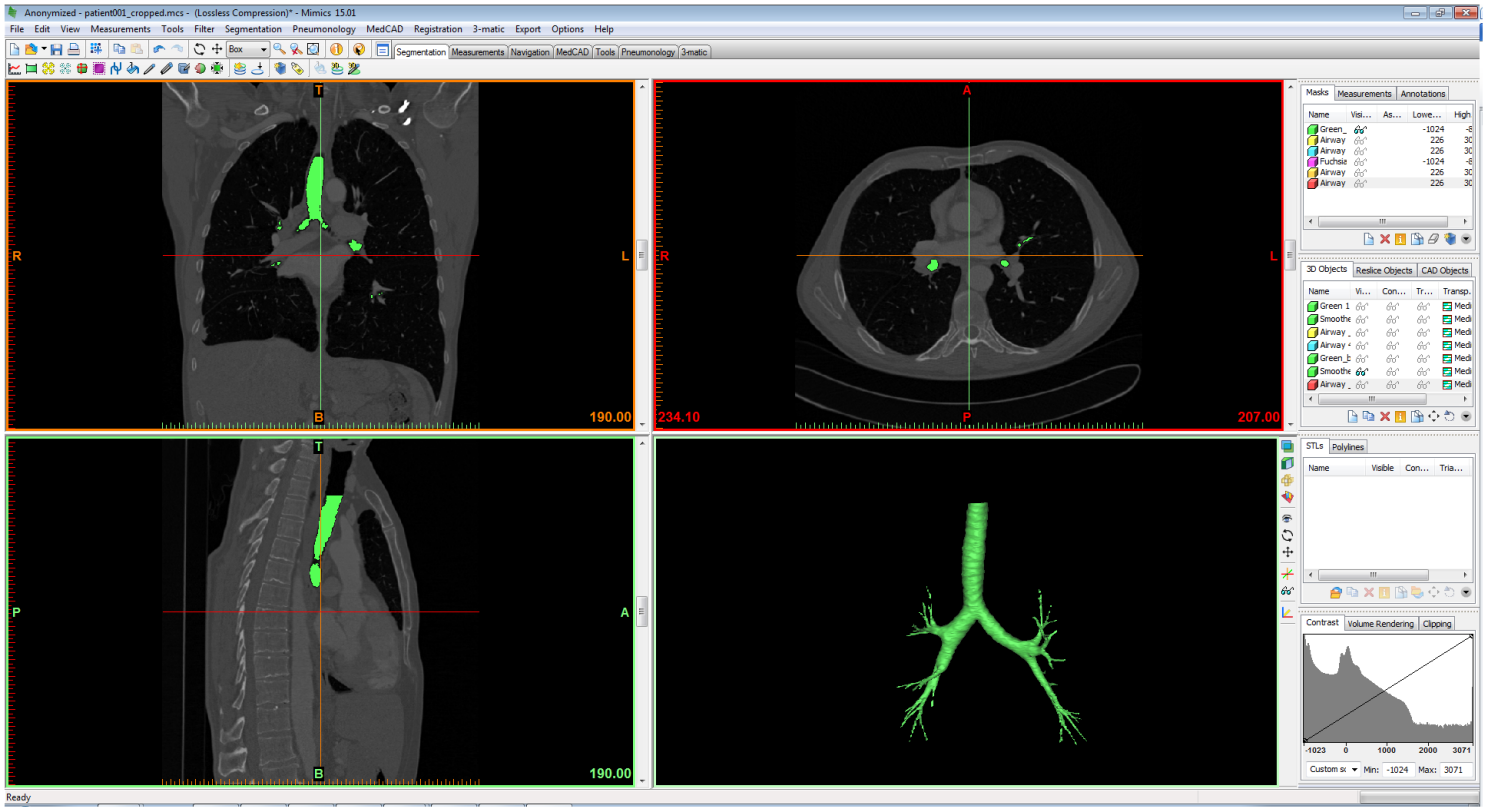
Mimics Graphical User Interface (GUI). In green the result of the segmentation with “Dynamic region growing” in MBM. A coronal, B axial, C sagittal view of the CT data and D the 3D model of the airway.
